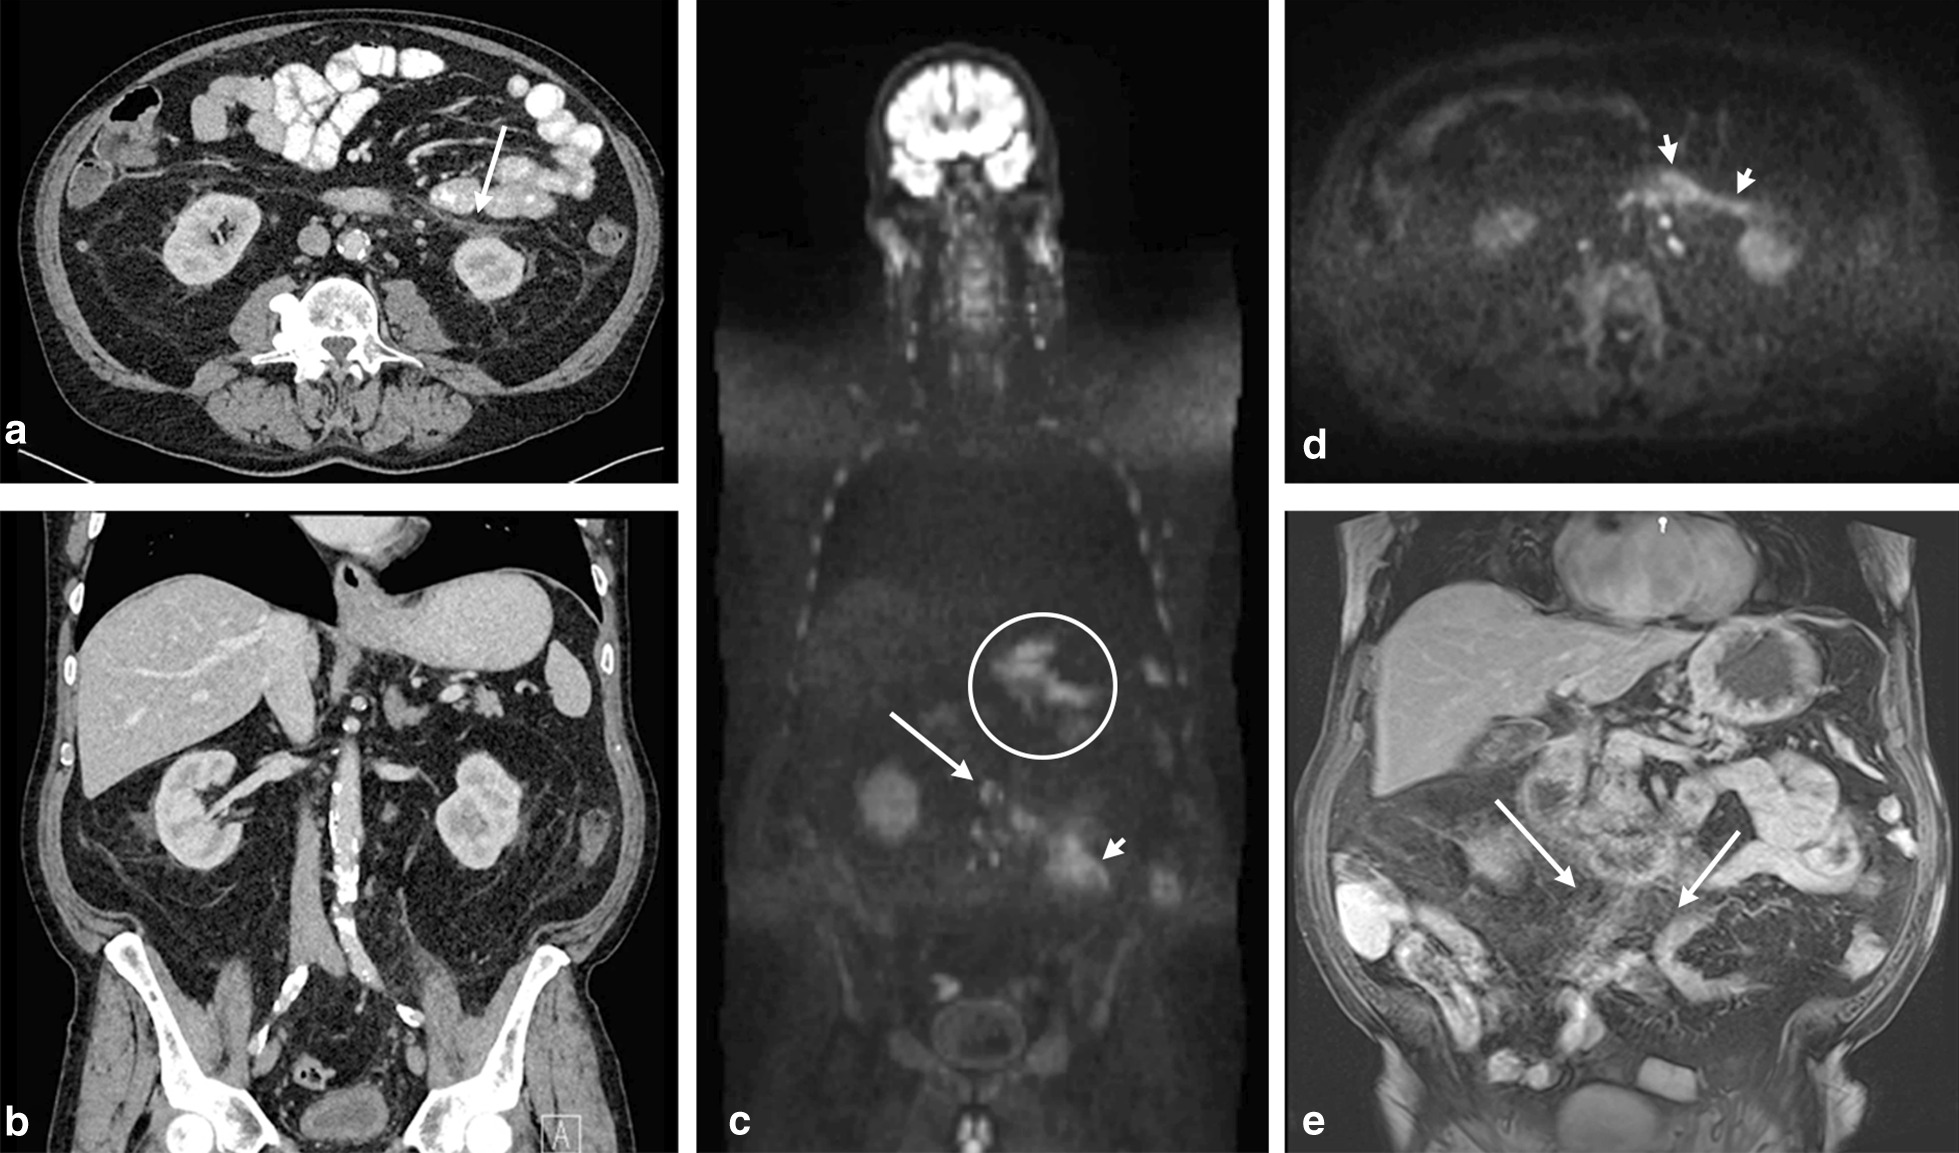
A patient with an age-range between 70 and 80 years old was diagnosed with primary gastric cancer. CT scan in the axial plane (a) with coronal reconstruction (b) did not show distant metastases. However, a slightly thickened anterior pararenal fascia on the left side (a, arrow) was noticed. WB-DWI/MRI was performed for further investigation with coronal (c) and axial b1000 (d) as well as contrast-enhanced T1-weighted images (e), and revealed –apart from the primary tumor (circled)– mesenteric tumor spread along the mesenteric root (c–e, arrows), as well as peritoneal tumor spread on the left anterior pararenal fascia (c, e, arrowheads)
